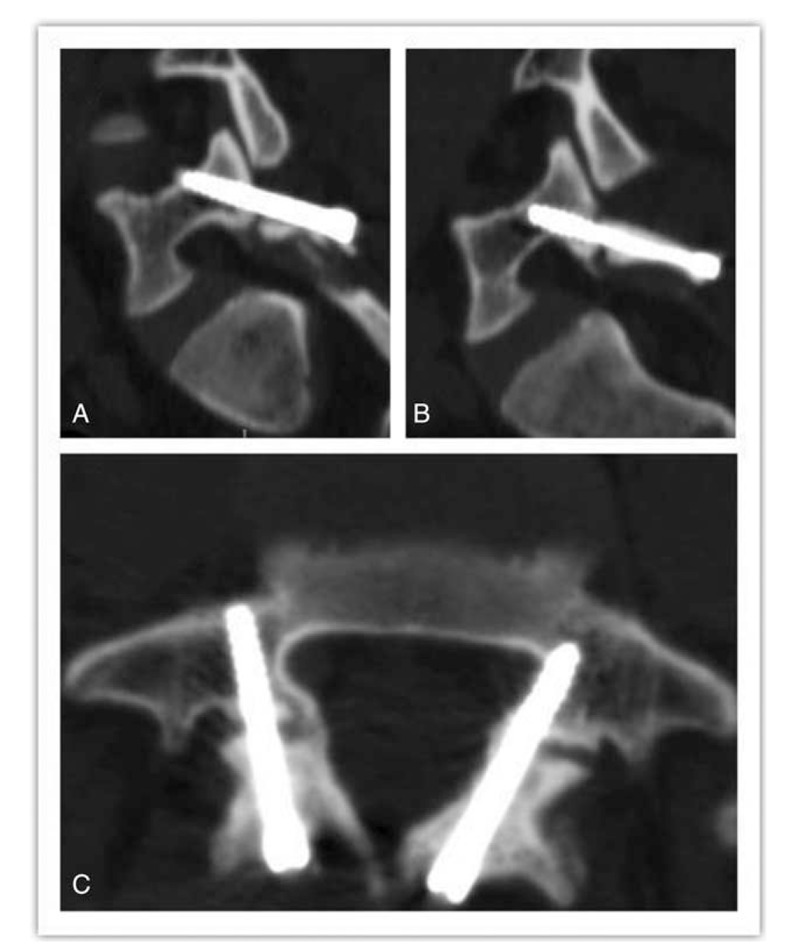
Axial and sagittal postoperative computed tomography images of the patient.
